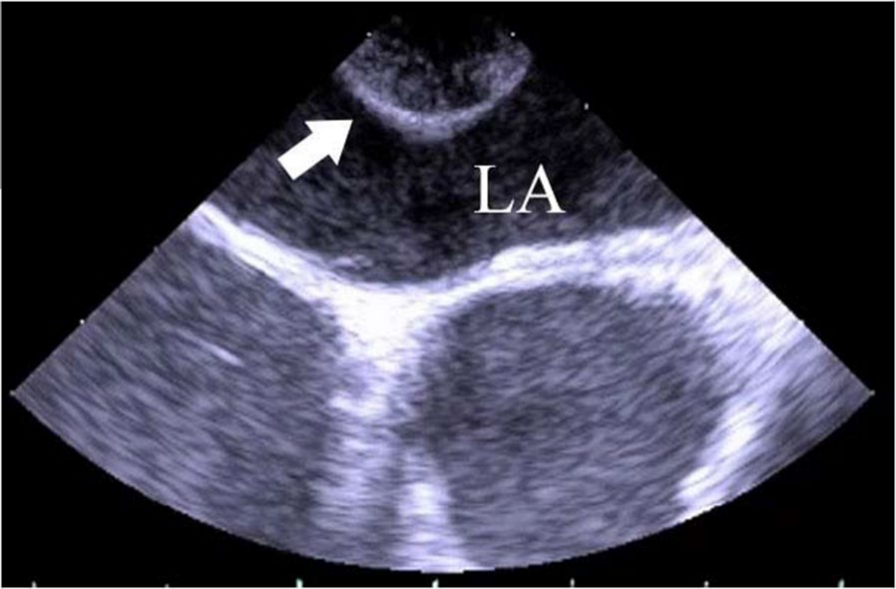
Transesophageal echocardiogram. Transesophageal echocardiogram shows the mass (arrow) projecting into the left atrium
